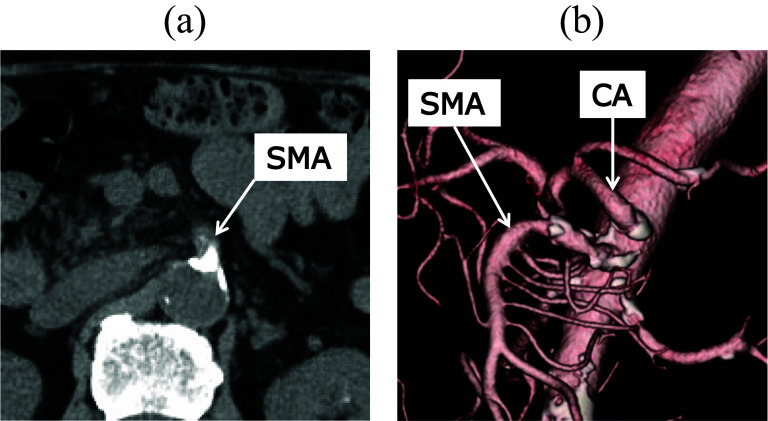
a Computed tomography (CT) shows superior mesenteric artery (SMA) stenosis due to atherosclerosis. b Three-dimensional image reconstructed from dynamic CT also shows SMA stenosis
